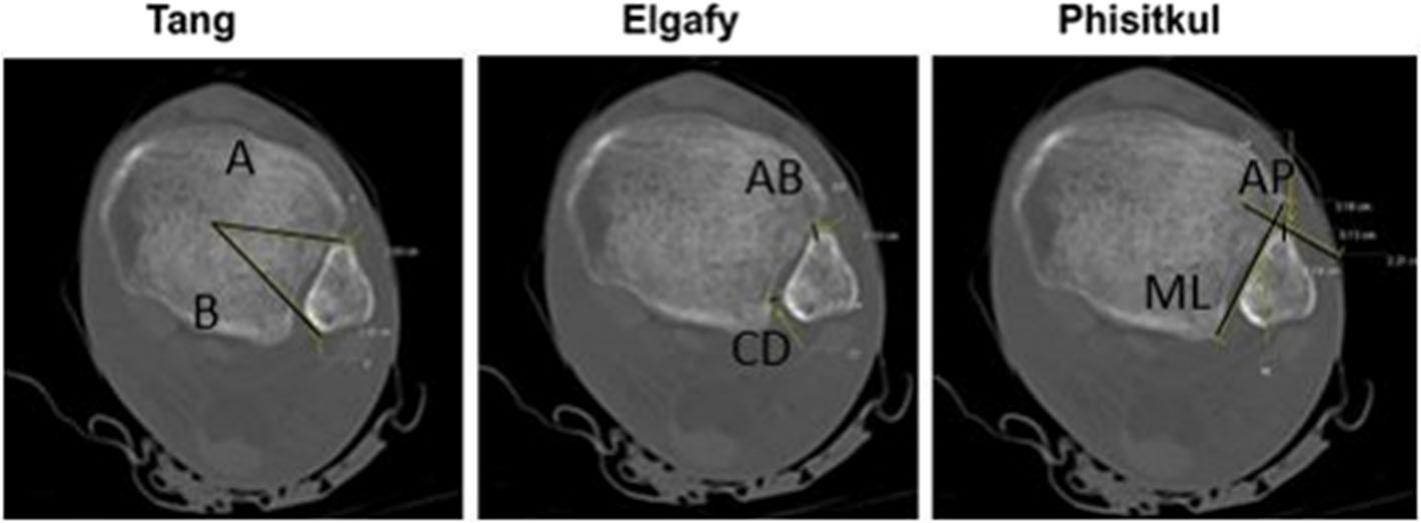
UltraVisual imaging software (Emageon, Inc., UltraVisual Medical Systems, Birmingham, AL) was used to perform measurements on the axial computed tomography scan view at 1 cm above the distal tibia articular surface. Tang et al. [23], ratio of A:B distance reflects fibular rotation. Elgafy et al. [24] and Phisitkul et al. [25] methods, direct measurements of anterior (AB and AP) and posterior (CD and ML) tibial-fibular distance, represent fibular translation in the coronal and sagittal planes, respectively
